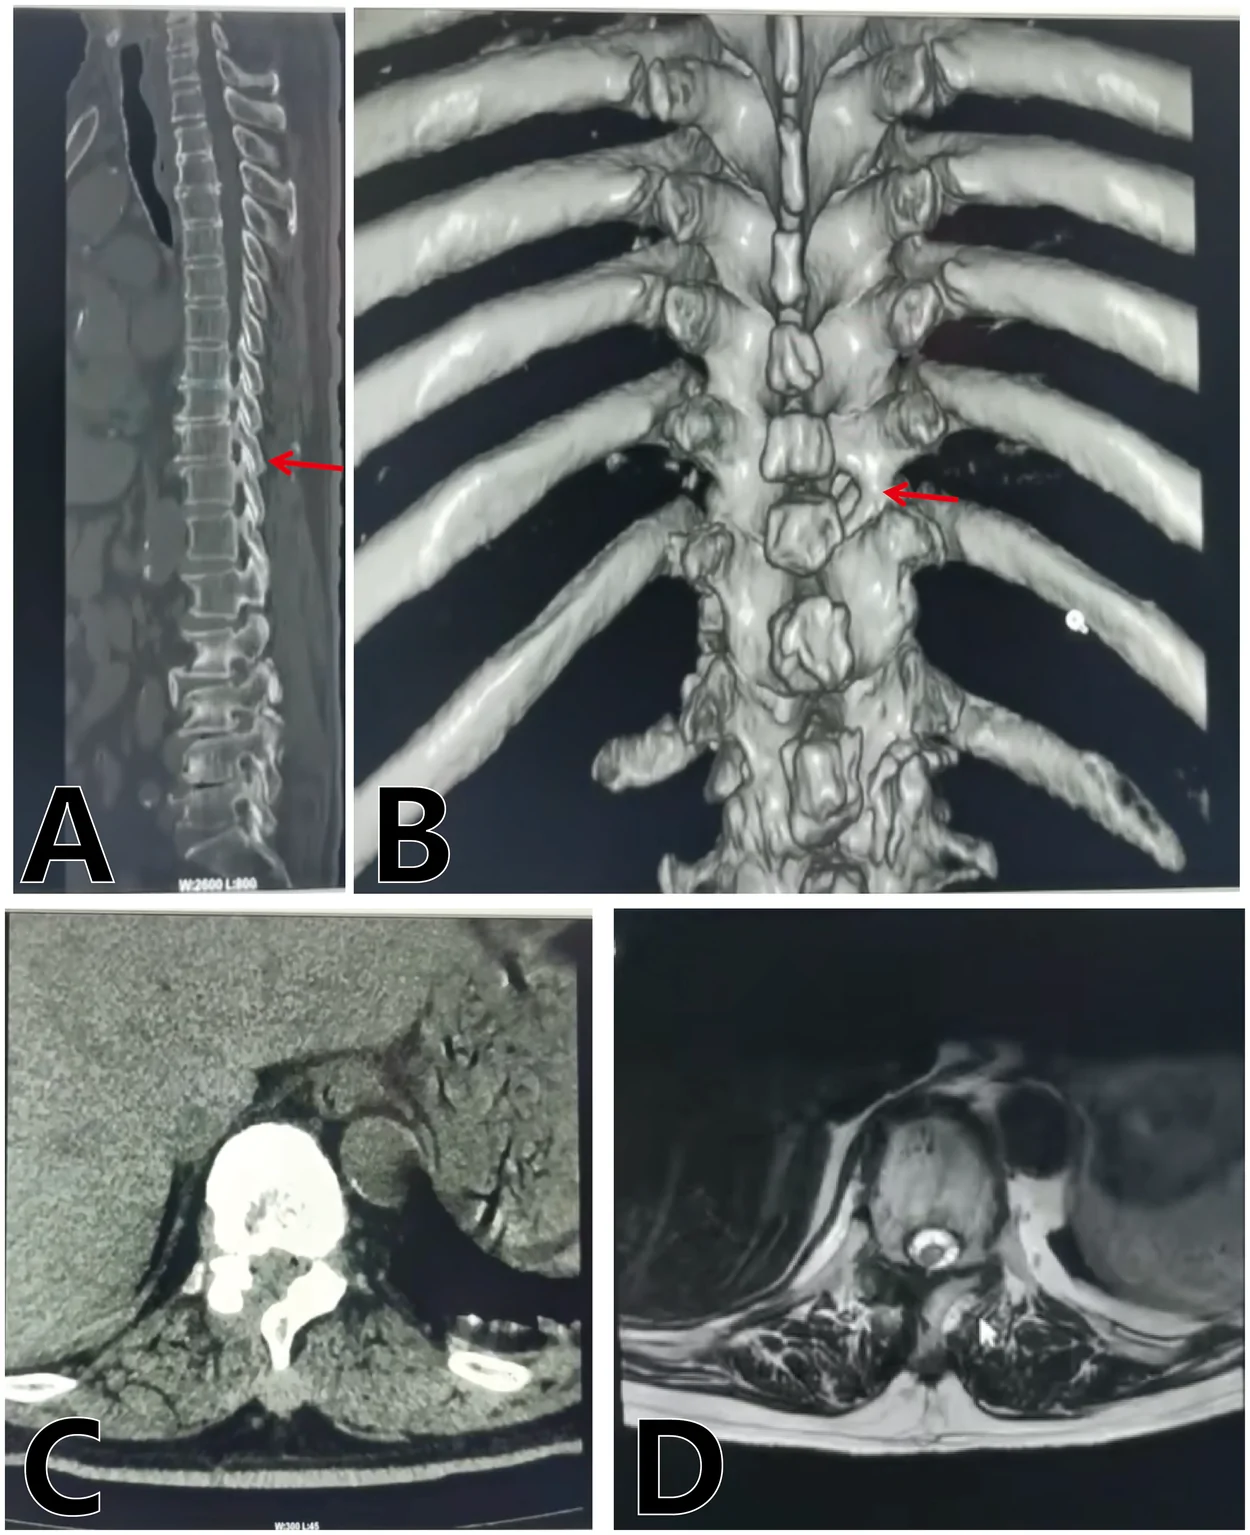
5-months post-operation images. (A–C) CT showed no evidence of postoperative vertebral instability. (D) MRI demonstrated recovery of spinal cord distension. Red arrows: herniated segment. CT, computed tomography; MRI, magnetic resonance imaging.
